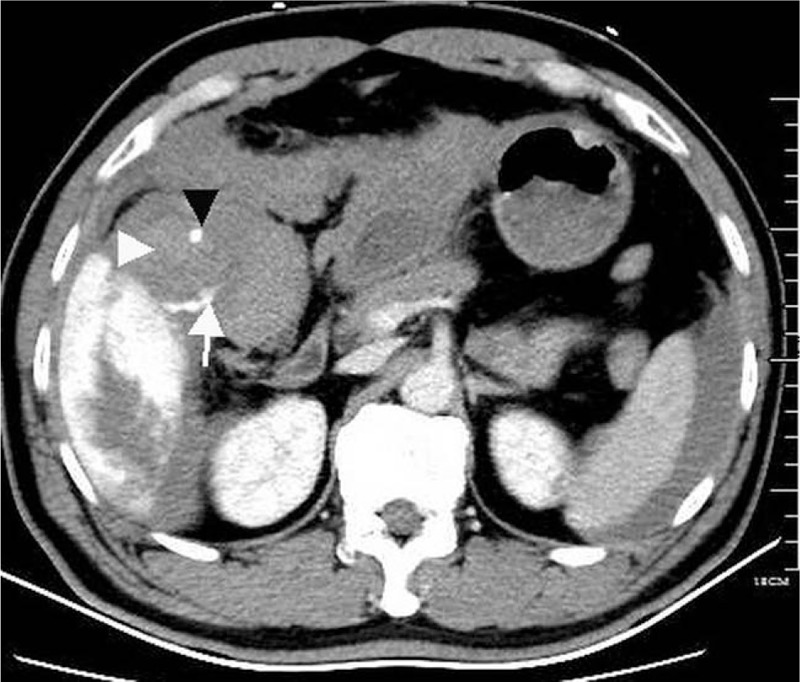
CT shows clots (*white arrowhead*) around a gallstone (*black arrowhead*) in the gallbladder and contrast extravasation from the hemangioma to the gallbladder (*white arrow*). CT = computed tomography.
